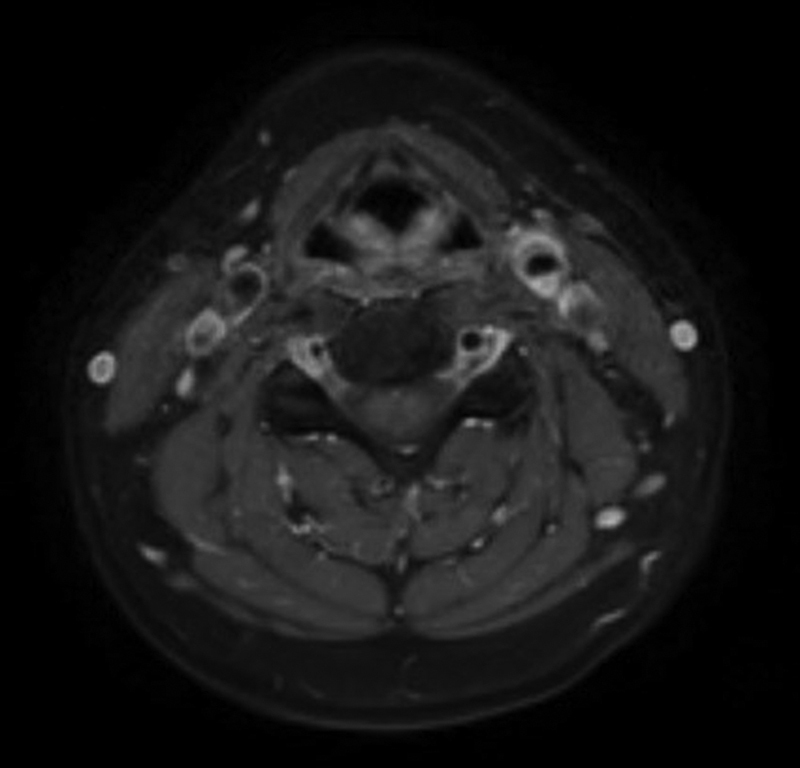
Magnetic resonance imaging at diagnosis.
